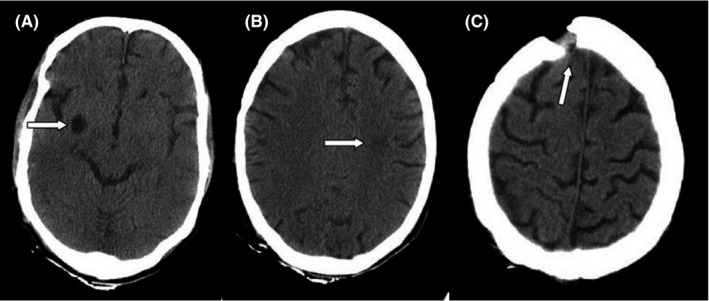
Computed tomography (CT) scans of the brain of the patient. A hypodensity in the left peritoneal (A), CSF‐like density in the right basal ganglia (B), a bone defect in the right frontal (C)
